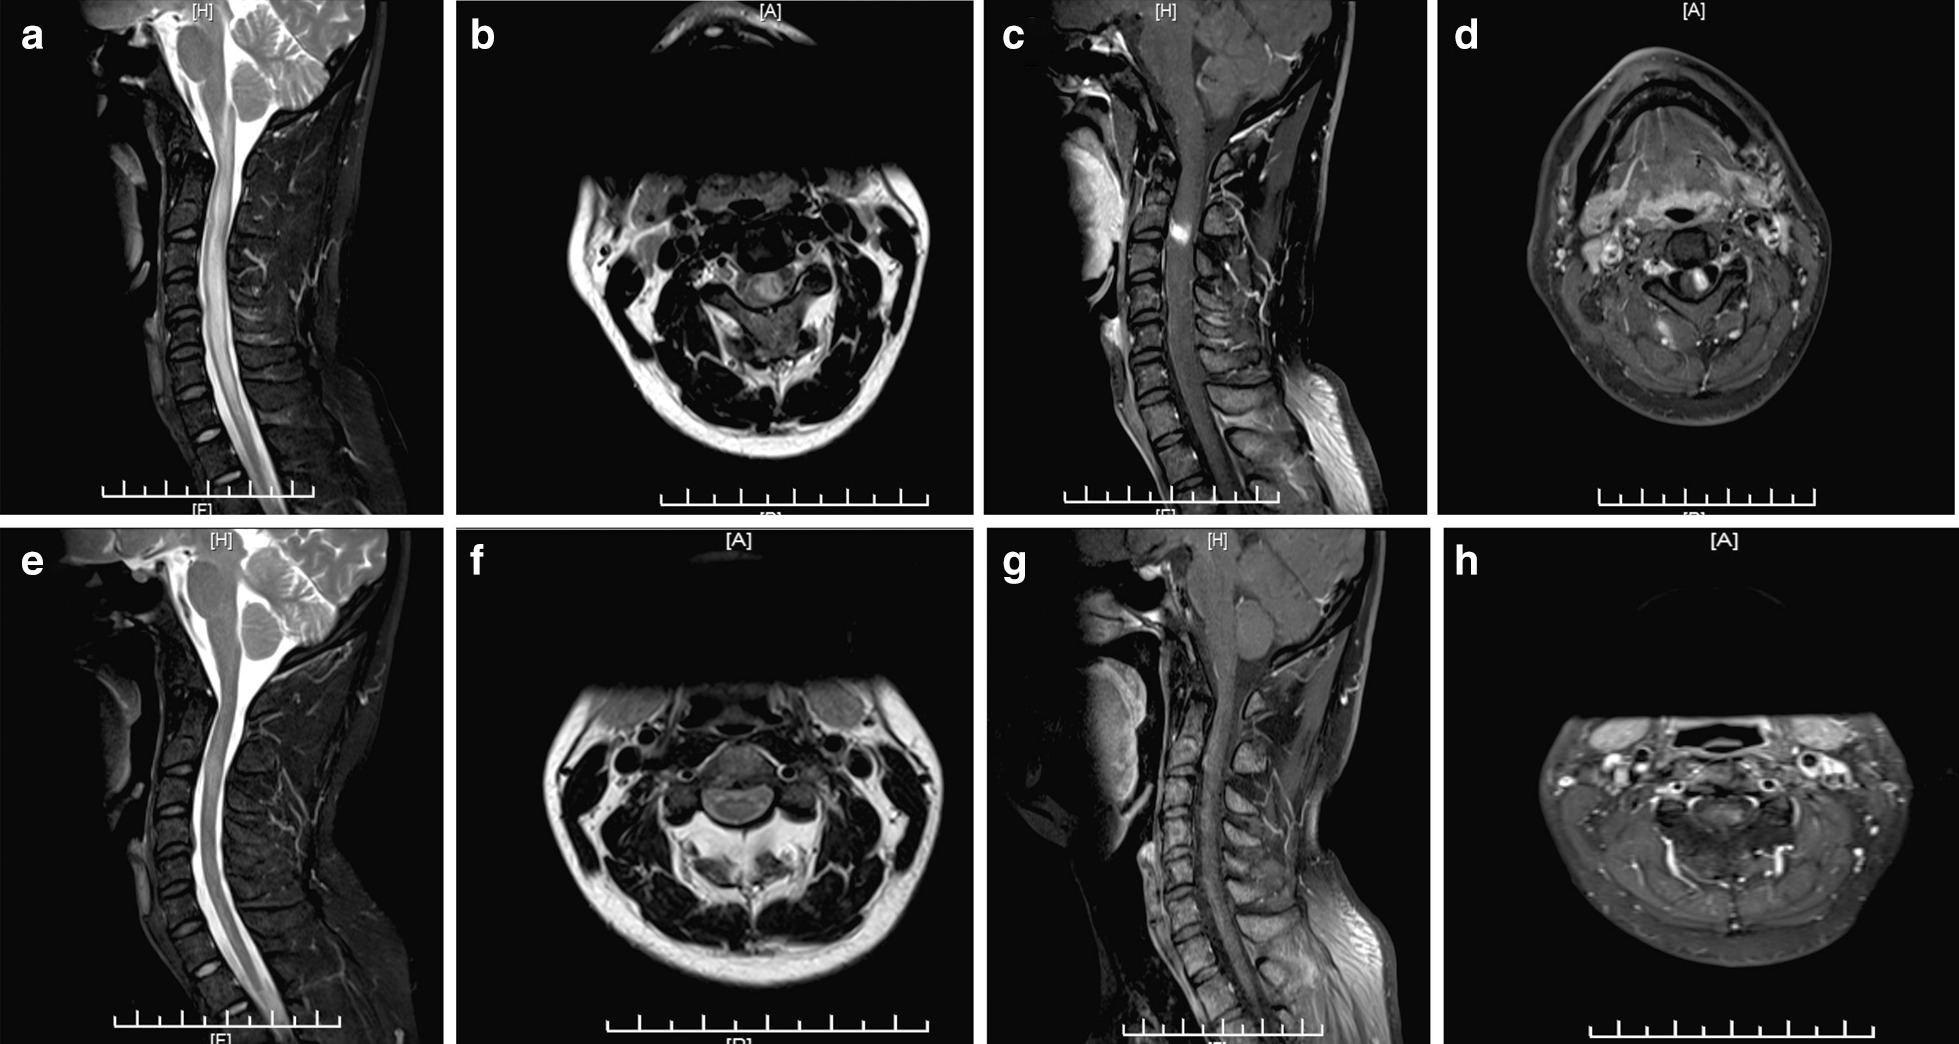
a–d Cervical magnetic resonance imaging (MRI) shows hyperintense signal changes from the medulla to the cervical cord with long segments in the T2-weighted image and enhanced signals from C2 to C3 in the T1-weighted image: a midsagittal T2-weighted image, b axial T2-weighted image, c midsagittal contrast-enhanced T1-weighted image, d axial contrast-enhanced T1-weighted image. e–h After treatment, cervical MRI shows that the swelling was reduced and that there were fewer enhanced lesions in the C2-3 region
